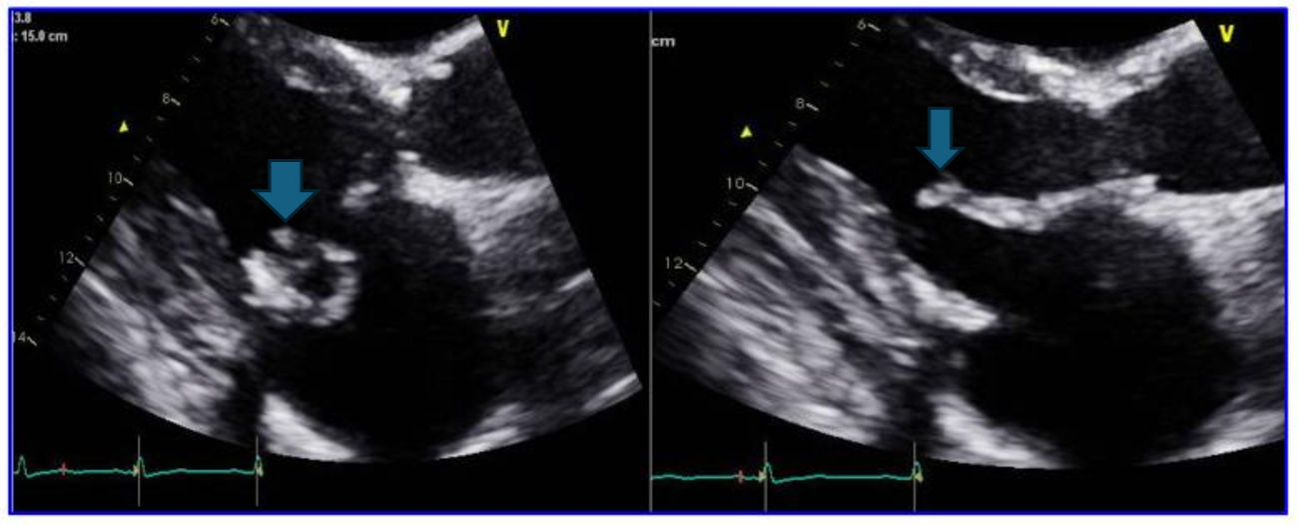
Transthoracic echocardiography pictures in the parasternal long axis view (PLAX) depicting a filamentous structure measuring 1.5 cm noted on both mitral valve leaflets, as indicated by blue arrows.
